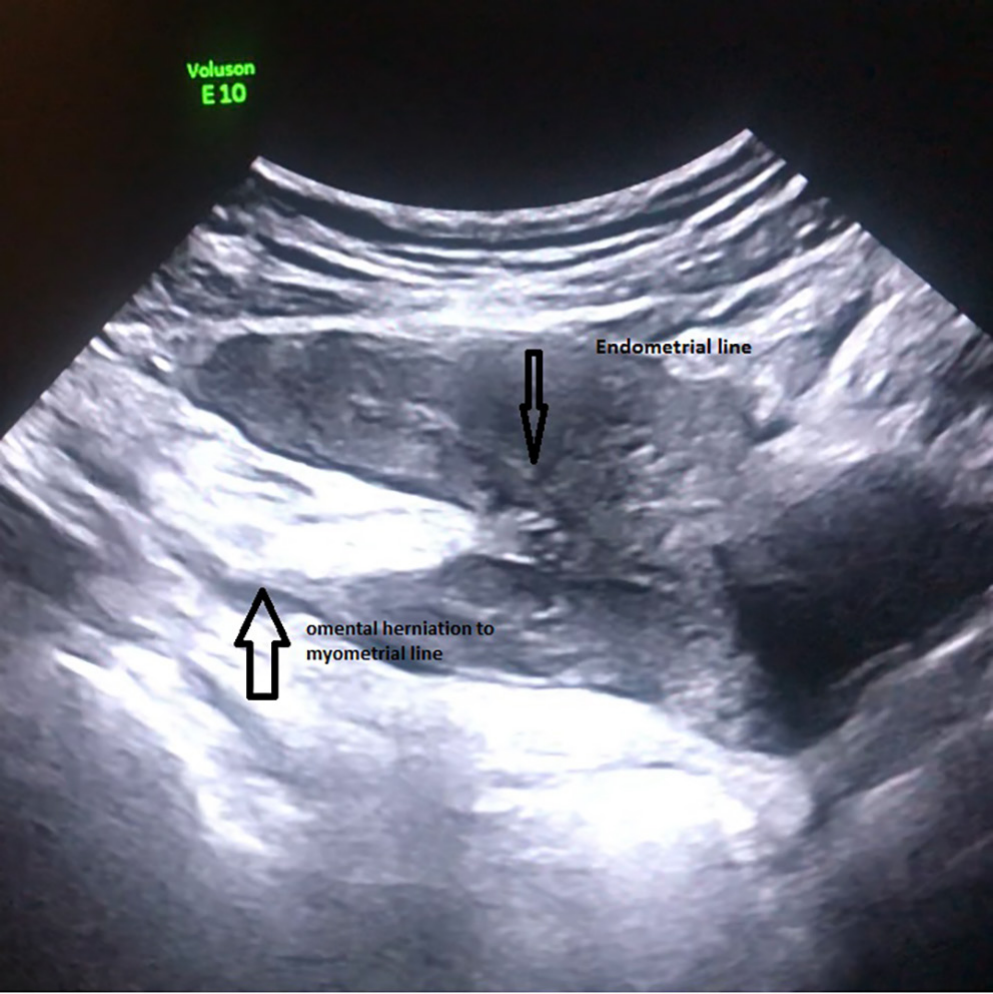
Transabdominal ultrasonographic image of the patient before the operation.
